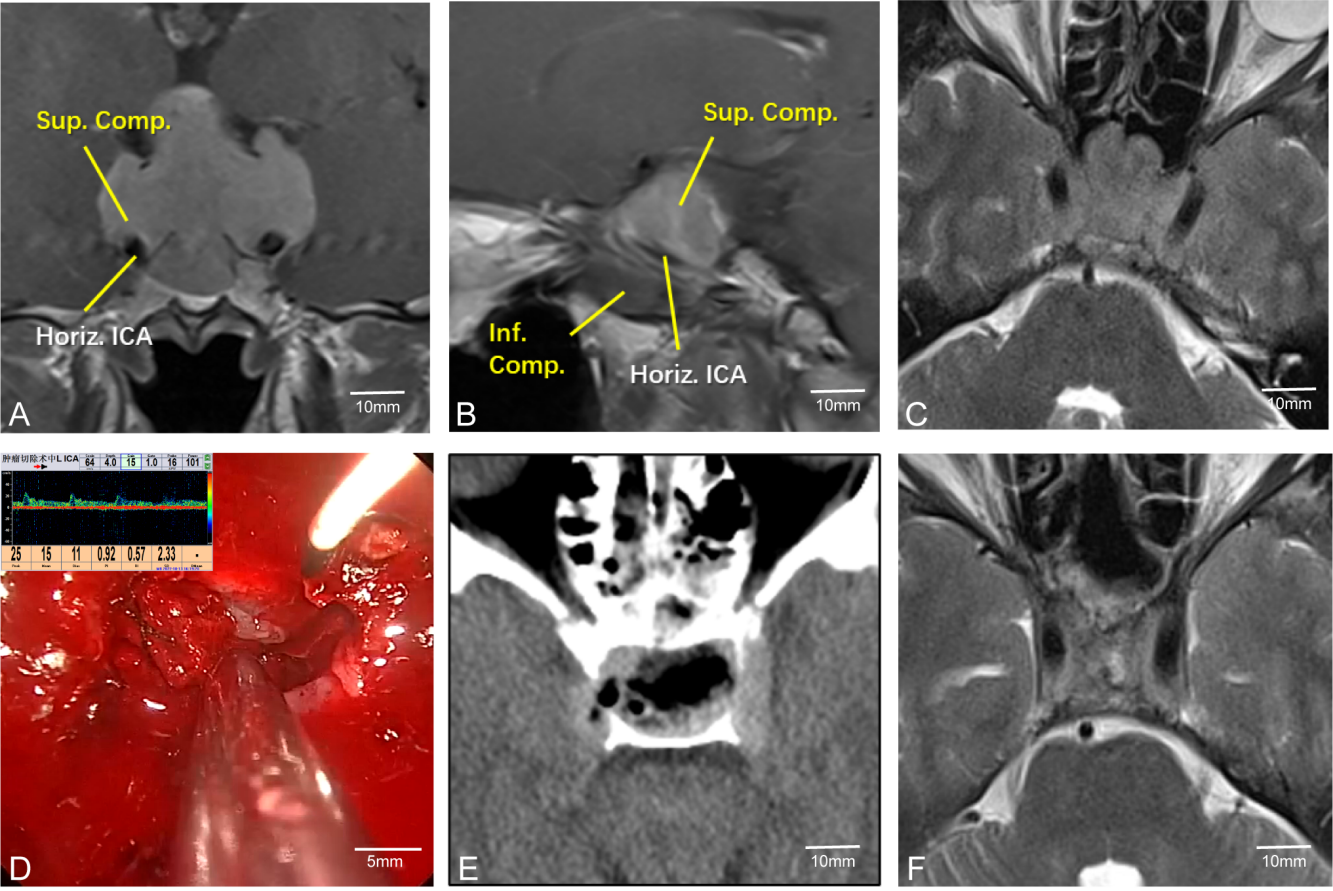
Case 24. Endoscopic resection of complex pituitary adenomas assisted by MVD. (A–C) Preoperative MR Showed that the left ICA was surrounded by the tumor. (D) Intraoperative determination of the position of the left internal carotid artery and the direction of blood flow. (E, F) Postoperative CT and MR demonstrated that the tumor was resected satisfactorily and the blood flow of the internal carotid artery remained unobstructed.
